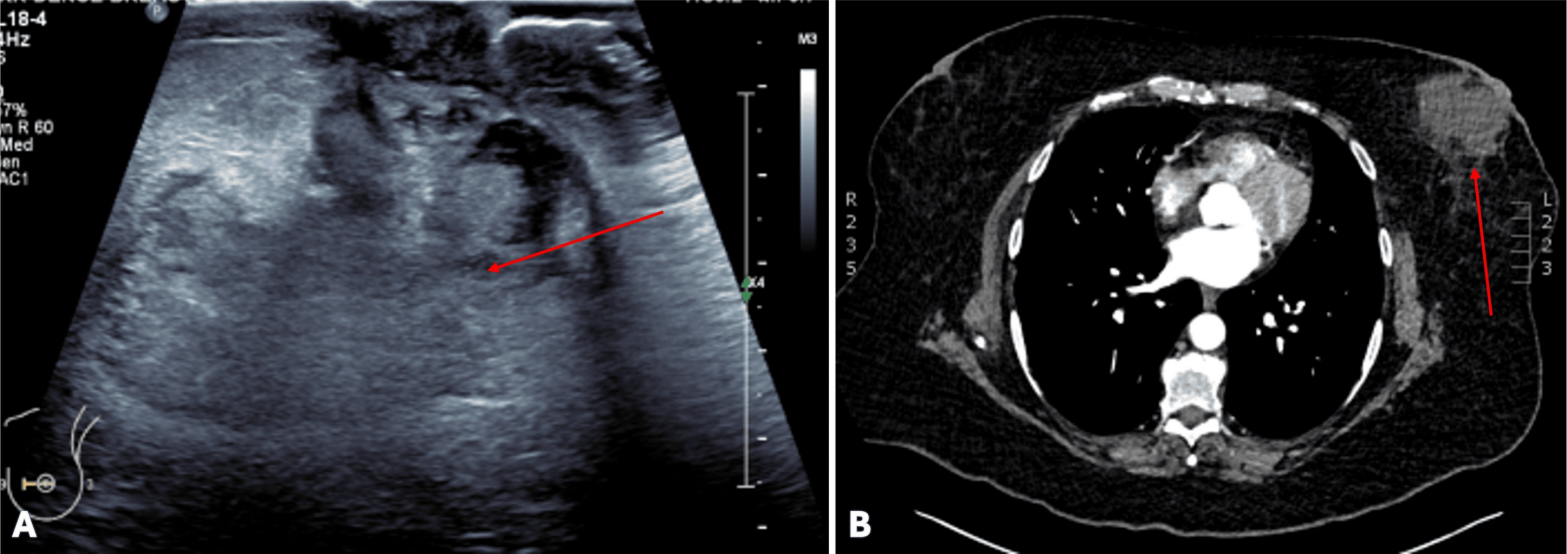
Ultrasound of the left breast and CT chest demonstrating left breast heterogenous collection consistent with a breast abscess (A: ultrasound image, B: CT chest axial view) CT: computed tomography
